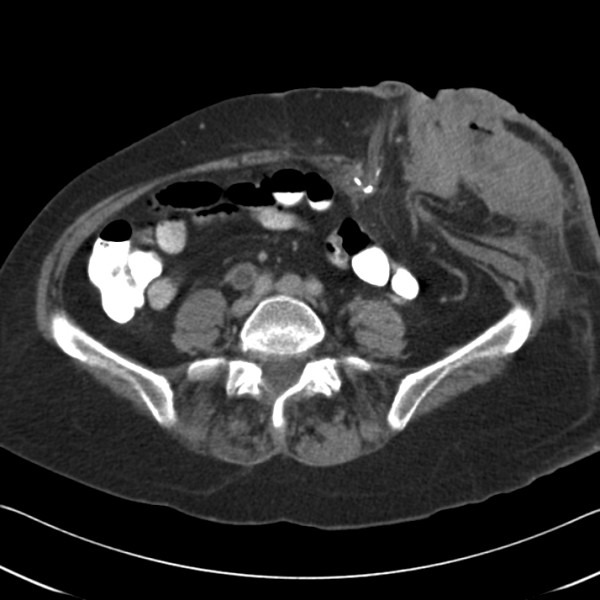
CT scan of the mass, showing abdominal wall metastasis.
